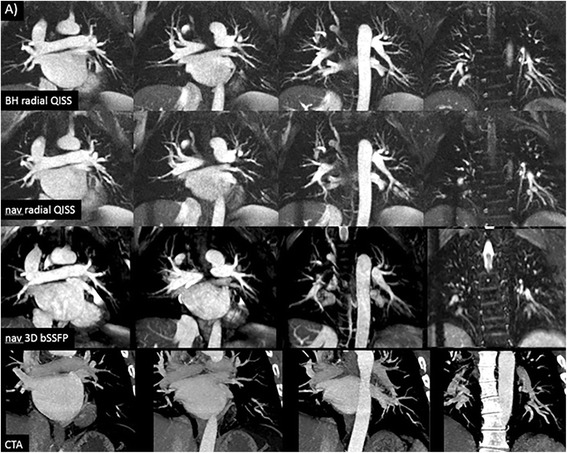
50-year-old male scheduled for pulmonary vein isolation, who was in sinus rhythm at the time of the CMR exam. a 12-mm thick maximum intensity projection images from coronal breath-hold radial QISS, navigator-gated radial QISS, navigator-gated 3D bSSFP, and CTA. Image quality is excellent with all MRA pulse sequences. Scan time was 3.4 min for navigator-gated QISS versus 10.3 min for navigator-gatd 3D bSSFP
